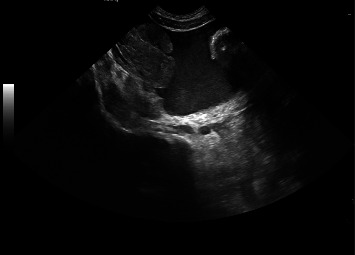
Abdominal ultrasound with hemoperitoneum.
